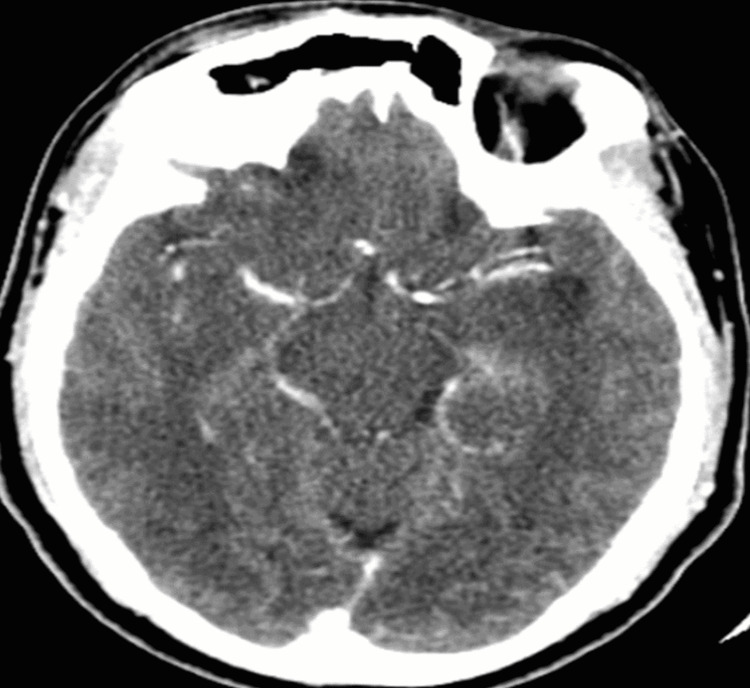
CT angiogram showing opacification of the left MCA post-administration of alteplase. MCA: middle cerebral artery
